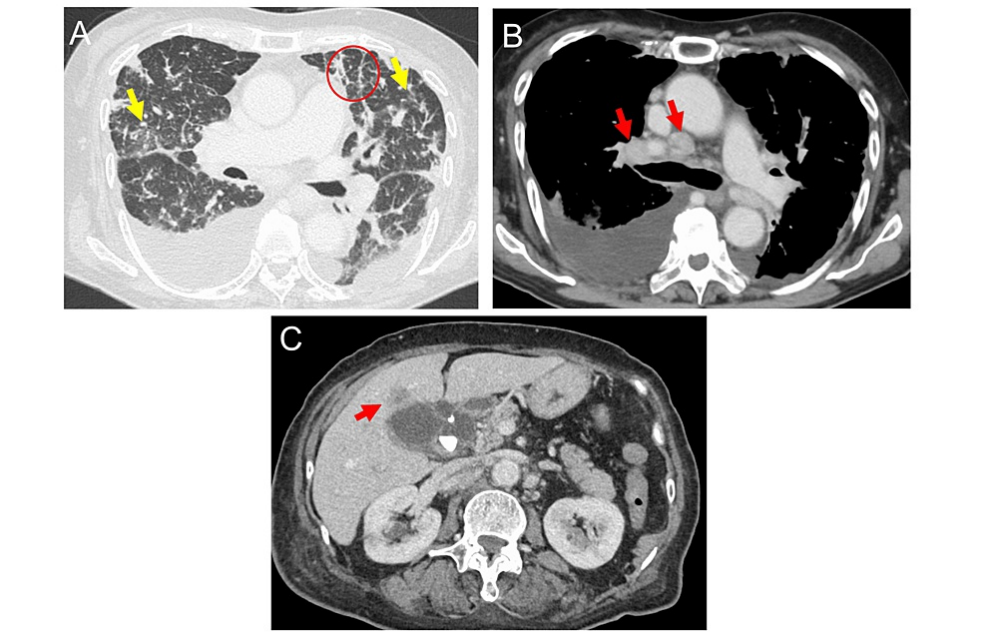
Computed tomography on admission A) The yellow arrows indicate multiple small nodular opacities.  The red circle indicates irregular thickening of the interlobular septa. B) Computed tomography shows further enlargement of mediastinal and hilar lymph nodes (red arrows) and appearance of bilateral pleural effusions. C) Computed tomography shows further enlargement of the liver mass. The red arrow indicates the liver mass. A layer is observed between the liver mass and the gallbladder.
